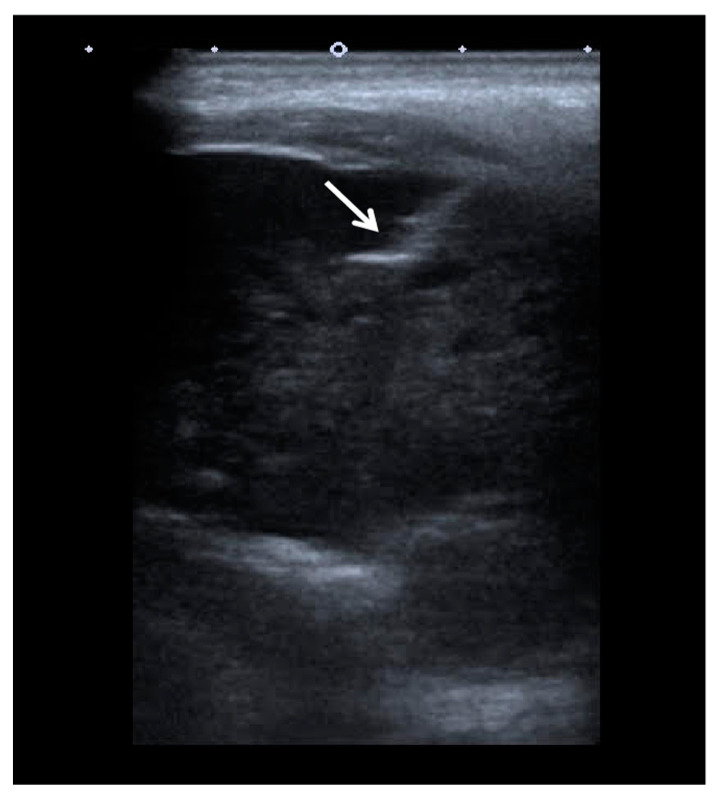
The ultrasound image of the left mandibular region mass obtained during the percutaneous ultrasound-guided core needle biopsy (arrow) shows the core biopsy needle inside the left mandibular region mass.
